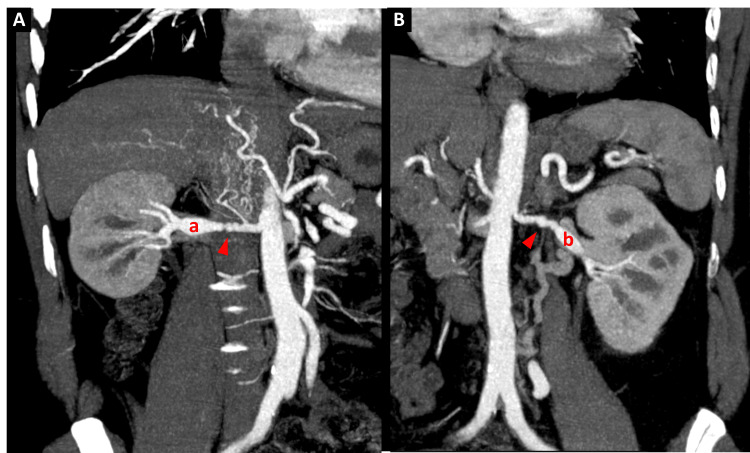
CT angiography of the renal arteries. Coronal reconstructions of the right renal artery (A) and left renal artery (B) demonstrated fibromuscular dysplasia with the characteristic “string-of-beads” appearance (arrowheads). a: right renal artery; b: left renal artery.
